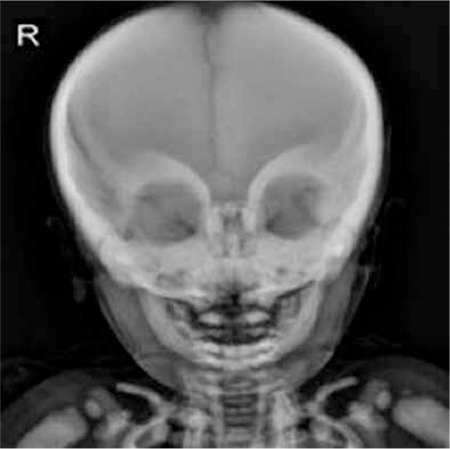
Radiograph of skull showing sclerosis and thickening of orbital rims.
